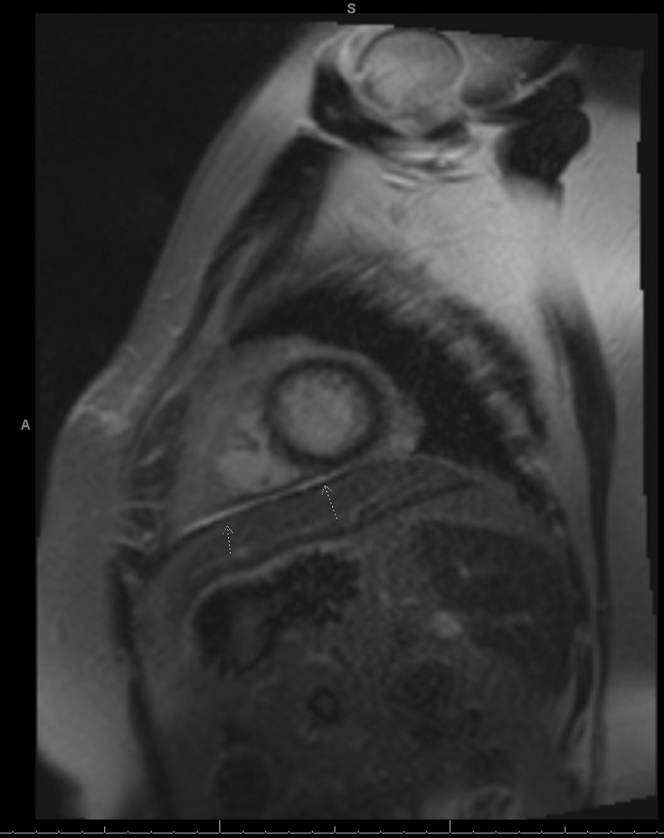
Cardiac Magnetic Resonance Imaging Cardiac magnetic resonance with thickened pericardium in the inferior aspect of the heart (arrow), with abnormal late gadolinium enhancement uptake.
